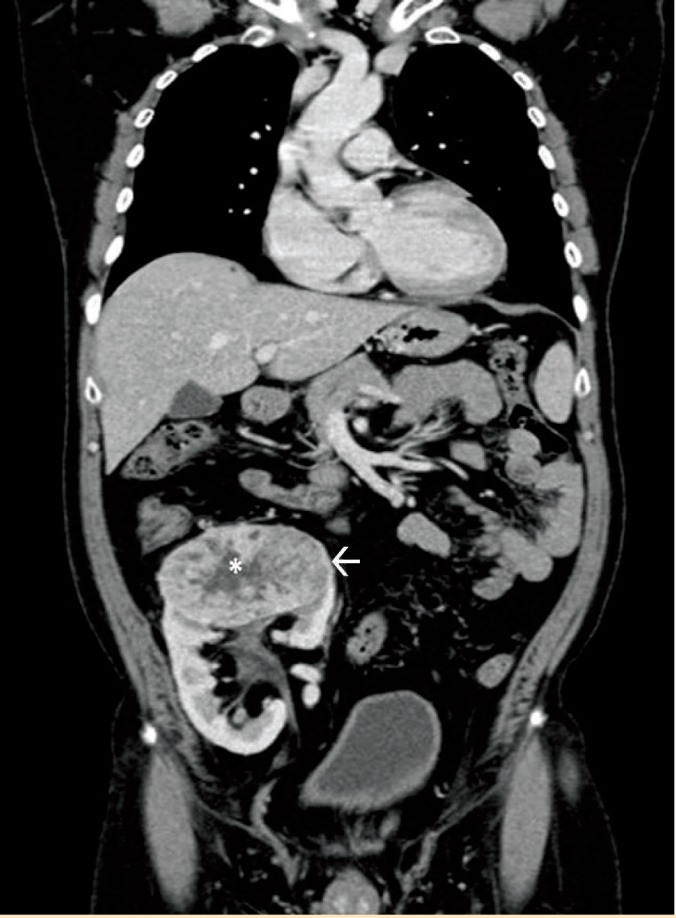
A 56-year-old man with renal cell carcinoma in renal allograft. Findings: Coronal CT with intravenous contrast, arterial phase of abdomen showing heterogeneously enhancing mass lesion at the upper pole of renal allograft (white arrow) in the right iliac fossa with internal hypodense nonenhancing region (asterisk) suggesting necrosis (Technique: Siemens Somatom sensation 64-slice CT scanner, Coronal CT, KV 120, Eff mAs 105, Slice thickness 5 mm. Contrast: Iohexol 350, 70 mL, Arterial Phase
